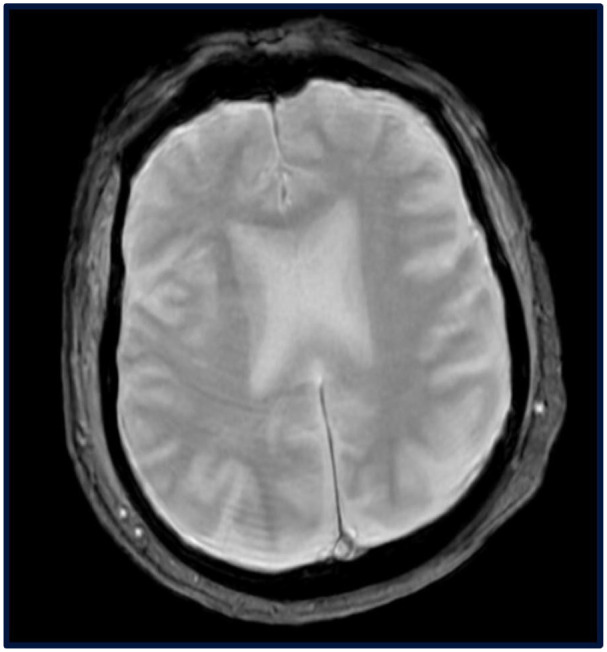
Brain MRI showing no acute infarct, abnormal signal changes, ventricular abnormalities, or extra-axial fluid collection. MRI, magnetic resonance imaging.
